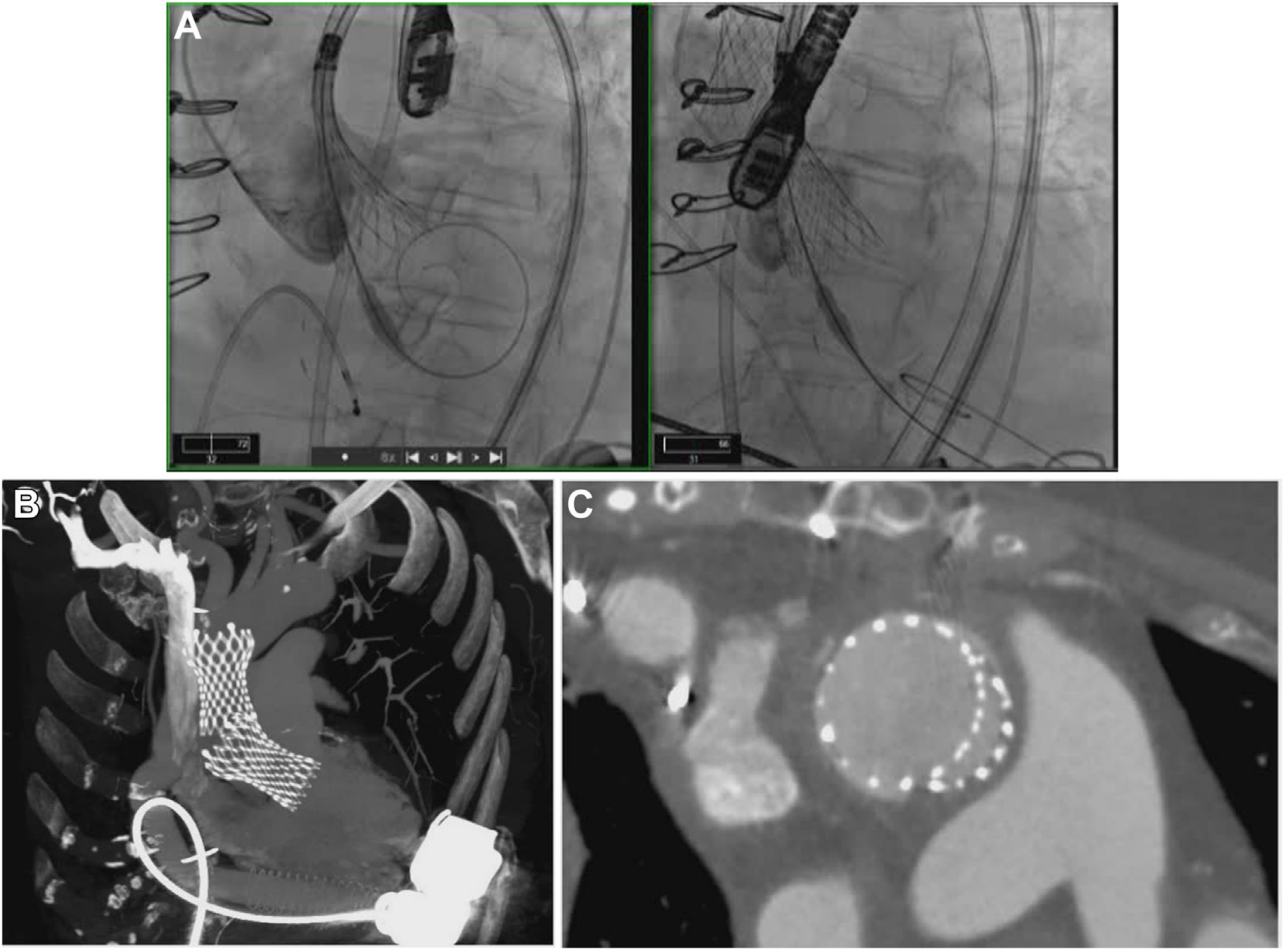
Patient 2: (A) Second Medtronic Evolut PRO+ deployed 4.0 to 5.0 mm below the annular plane. (B) Postprocedural computed tomography showing 2 stabilized Evolut valves. (C) Computed tomography short-axis view.
